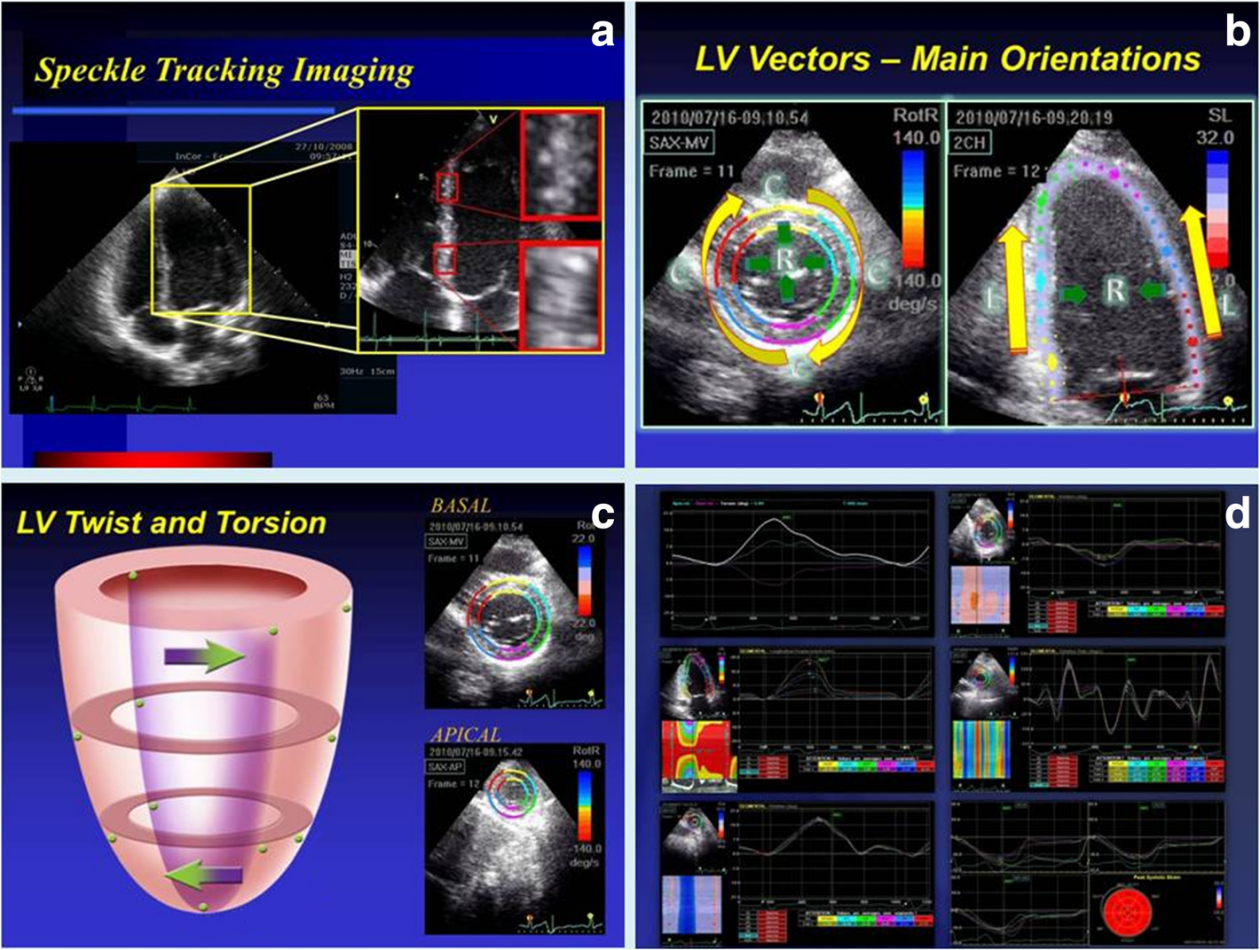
Speckle tracking echocardiography. Zoom image of myocardial ultrassonographic appearance, with the speckles (a). Main orientations of LV contration: R, radial; C, circumferential; L, longitudinal (b). Representation of LV twist – clockwise rotation at the basis and counterclockwise at the apex (c). Example of myocardial mechanics analysis (d)
